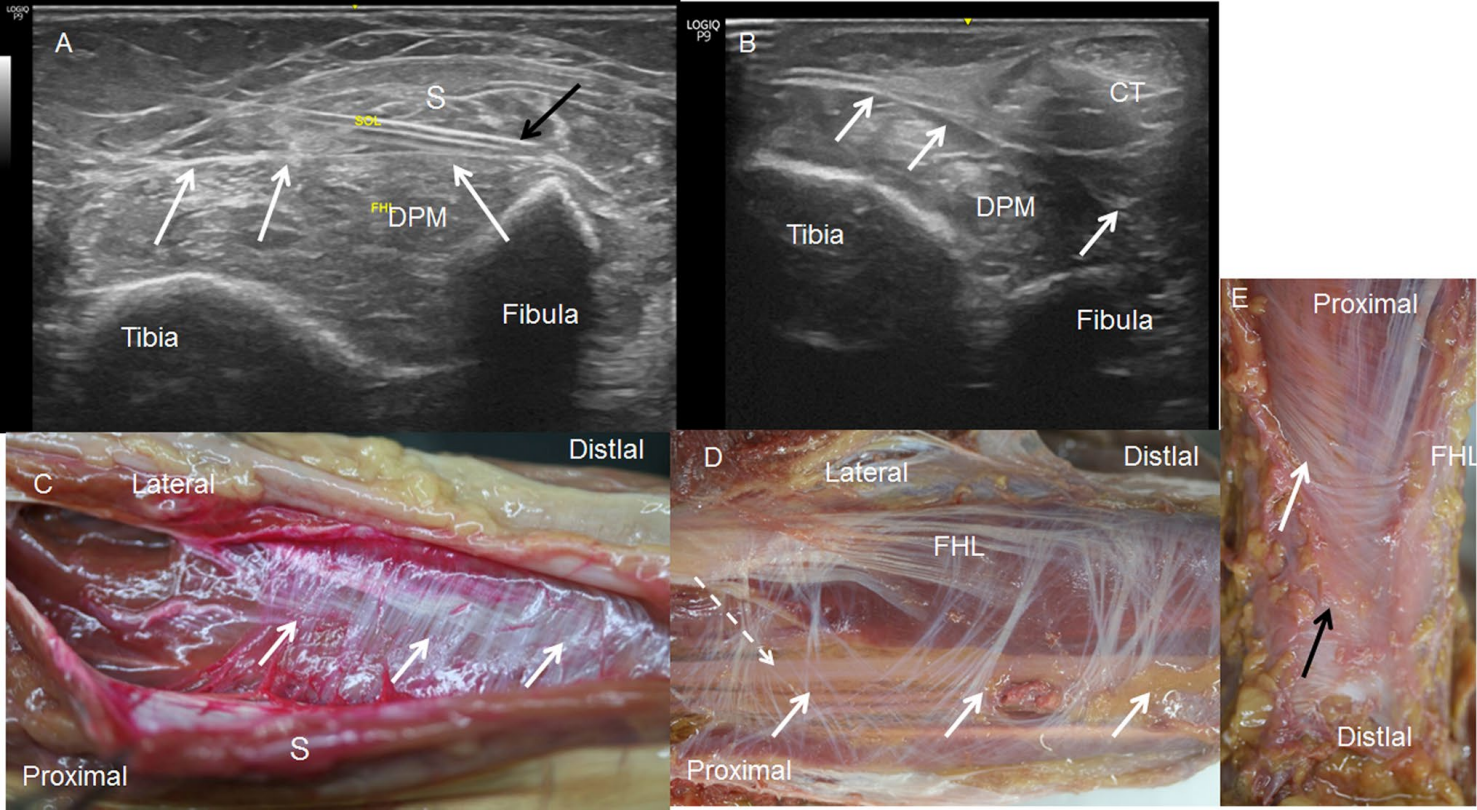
Posterior compartment of leg. A Ultrasound view of a right leg where the hyperechogenic line (white arrows) divided the deep posterior muscles of the leg (DPM) from the soleus muscle (S) The needle (black arrow) was under the soleus muscle to inject the dye. B The ultrasound view of the hyperechogenic line (white arrows) between the DPM and the calcaneal tendon (CT) at the inferior third of a left leg. C, D, E Anatomical view of the septum after the triceps surae muscle has been removed in all the examples. C Septum (white arrows) separates the deep posterior muscles of the leg from the soleus muscle (S). D Under the septum flexor hallucis longus (FHL) and tibialis nerve (white arrows) are identified. E The septum (white arrow) is thicker and more evident in the inferior part (black arrow) of the leg
